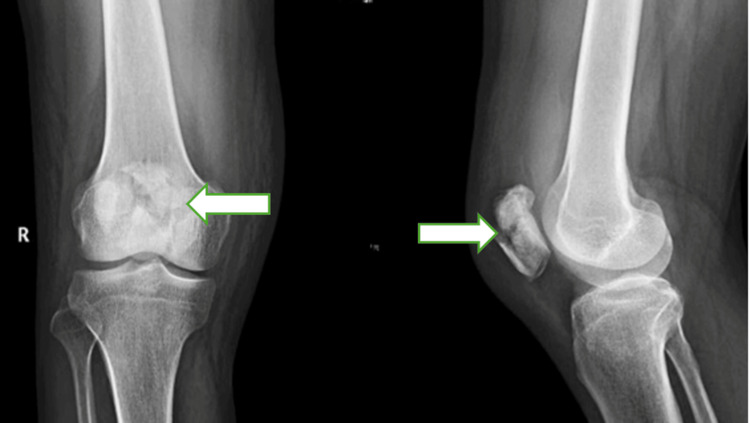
Preoperative radiograph (anteroposterior and lateral views) showing comminuted patella fracture (AO/OTA 34-C3) with multiple displaced fragments.
